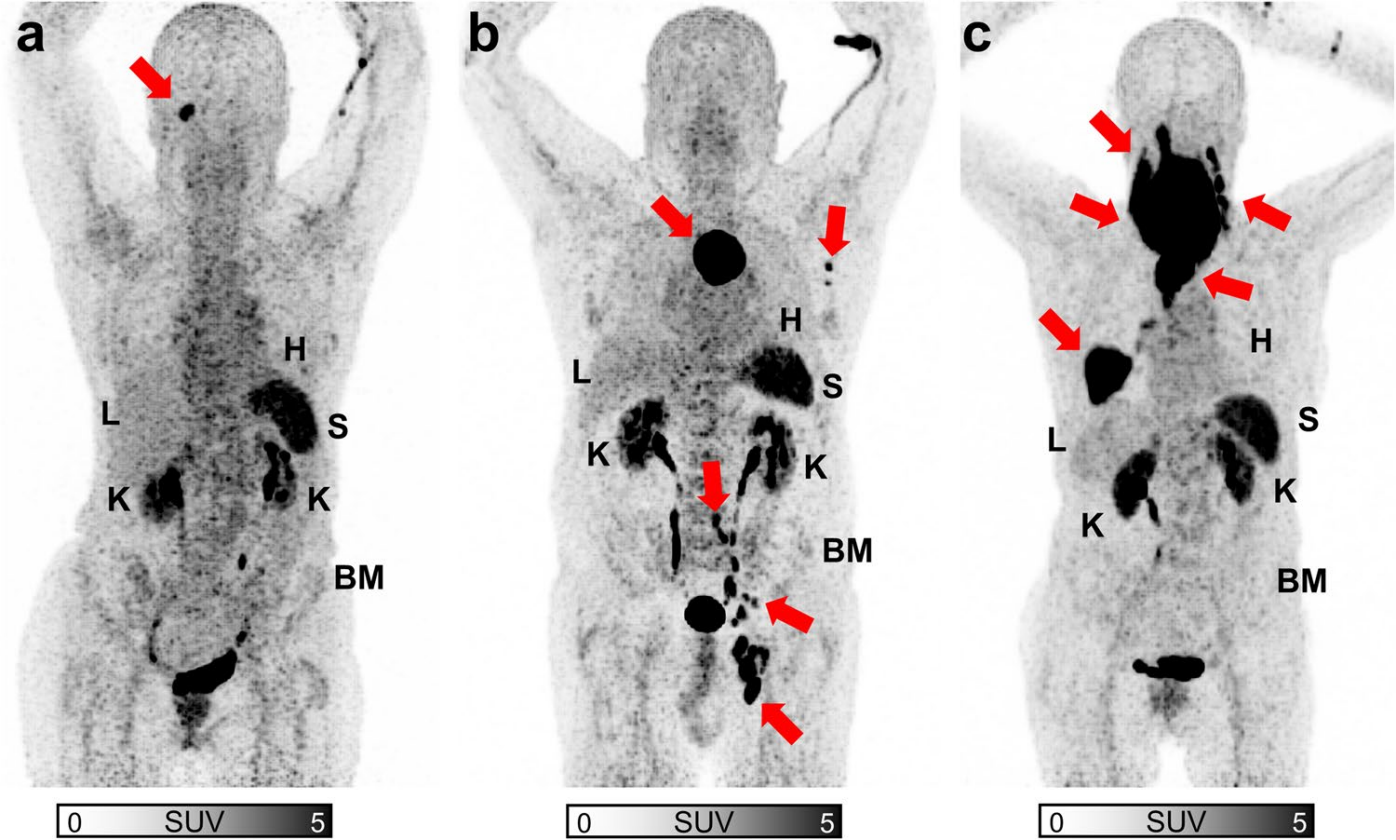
Maximum intensity projections of [^68^Ga]Ga-PentixaFor PET/CT in patients with low (a lymphoma volume, 1.7 cm^3^), intermediate (b lymphoma volume, 66.2 cm^3^) and high (c lymphoma volume, 361.5 cm^3^) lymphoma load. Red arrows indicate lymphoma manifestations. Visually, no obvious differences in normal organ uptake are apparent between different patients, supporting the notion that in patients with high lymphoma manifestation burden, uptake in normal organs does not drop. H = Heart, L = Liver, K = Kidney, S = Spleen, BM = bone marrow
